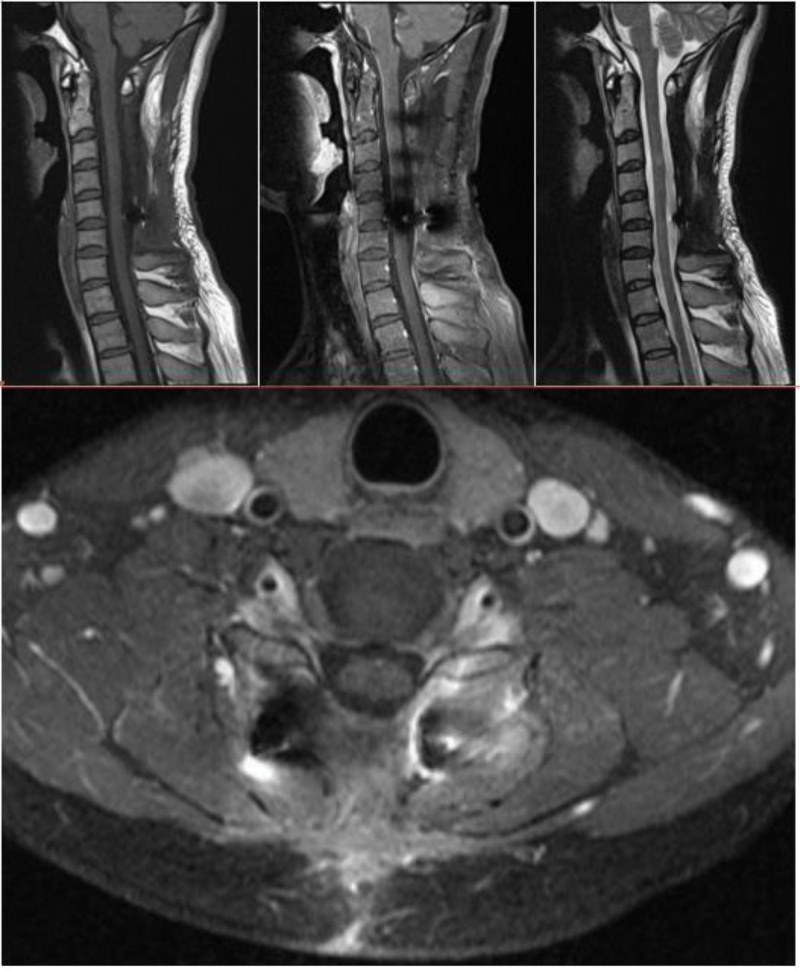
Second postoperative magnetic resonance imaging at 4 mo after surgery.
